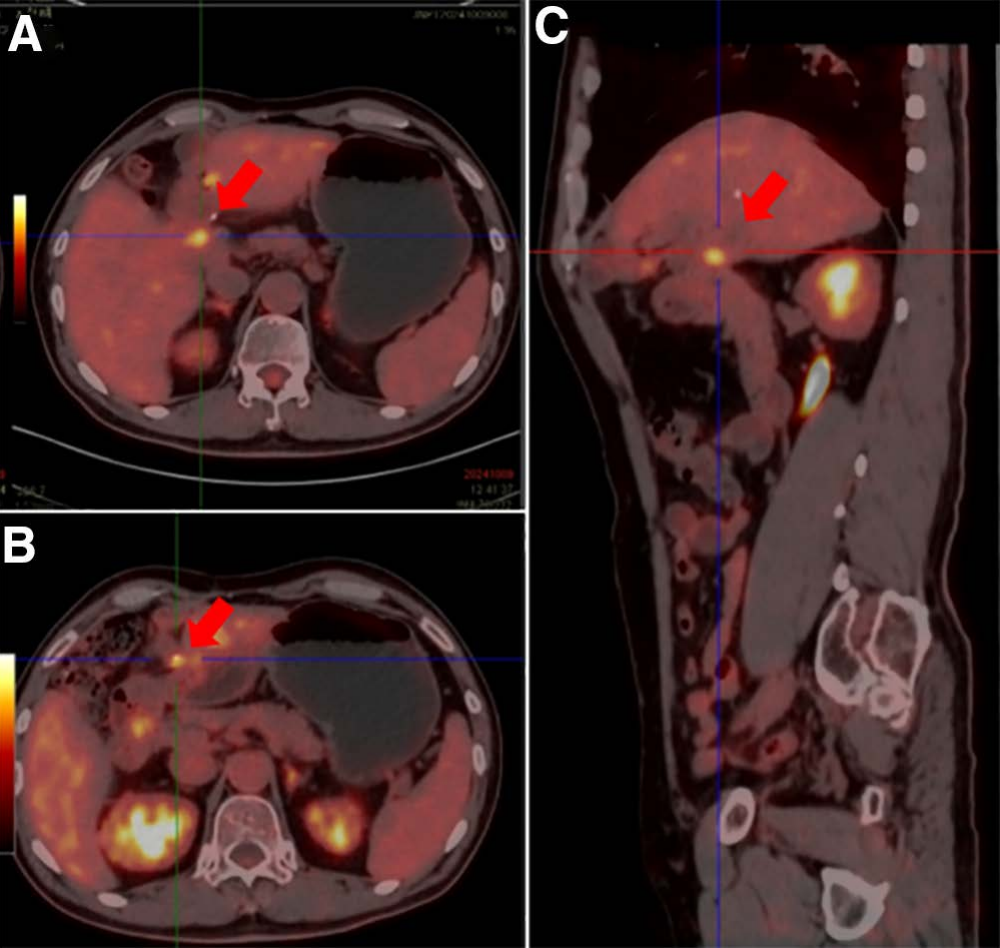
Axial (A and B) and sagittal (C) views at different levels. The positron emission tomography/computed tomography showed that the metastases were still showing tumor activity.
